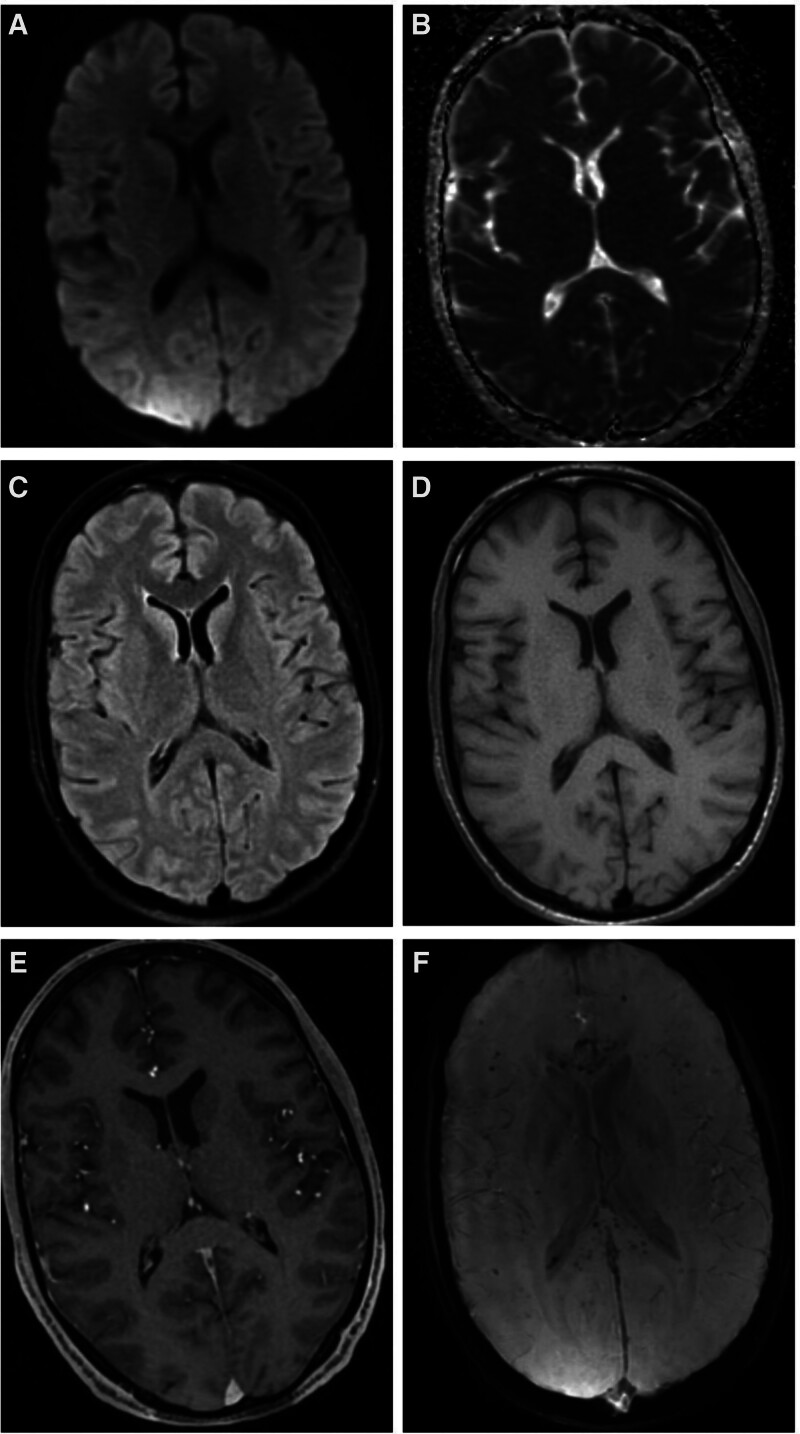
MRI of the brain (A) Diffusion weighted sequence and (B) apparent diffusion coefficient without any diffusion restriction. (C) Axial T_2_-weighted FLAIR sequence and (D) axial pre-contrast T_1_-weighted sequence. (E) Axial post-contrast T_1_-weighted sequence. (F) Susceptibility weighted imaging sequence showing juxtacortical hypointensities and significant hypointensities within the corpus callosum. FLAIR = fluid-attenuated inversion recovery, MRI = magnetic resonance imaging.
